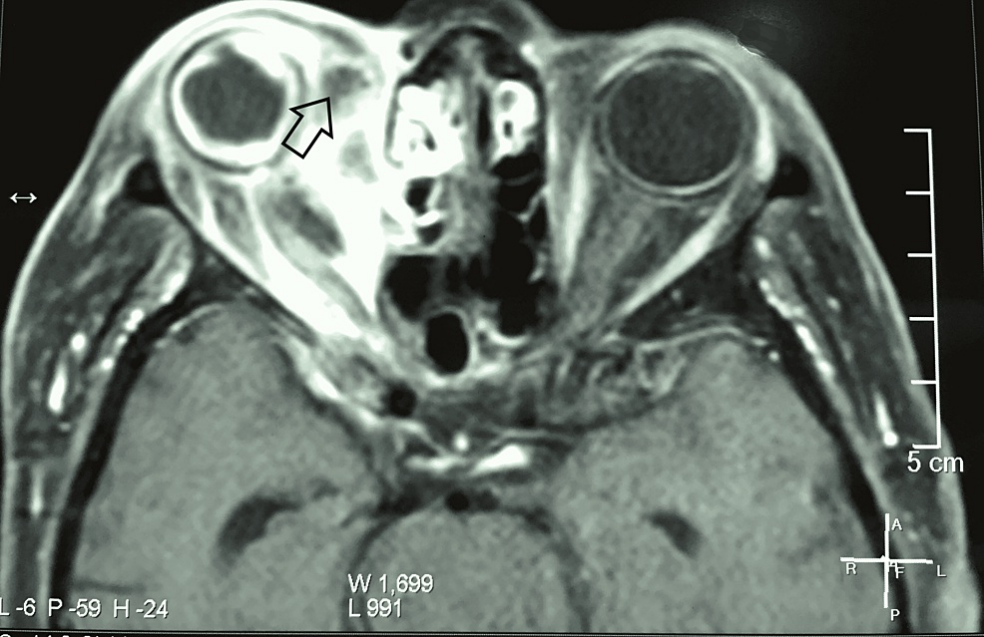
Magnetic resonance imaging of the brain and paranasal sinuses showing right-sided periosteal abscess formation
